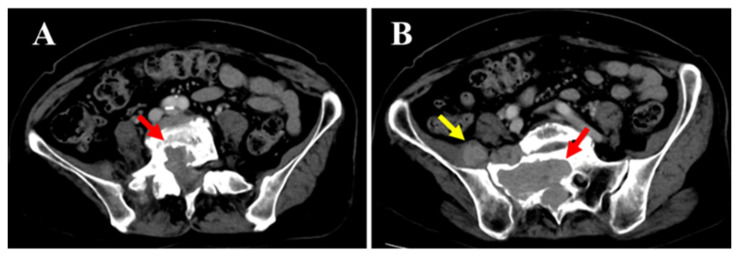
Spontaneous pain and numbness from the right buttock to the posterior thigh and lower leg worsened since the initial visit. Sensory dullness was mainly observed in the anterior lower leg and dorsum of the foot. The muscle strength in the lower limb was MMT grade 0 for both the tibialis anterior and extensor hallucis longus, while all other muscles remained at grade 5. Bladder or bowel dysfunction was not observed. Contrast-enhanced computed tomography (CT) (A,B the mass within the spinal canal (red arrow) and outside the intervertebral foramen (yellow arrow)) showed that the mass had increased in size over time and demonstrated bone invasion. Compressive changes resembling scalloping were also observed. No obvious metastasis to the other organs was observed.
